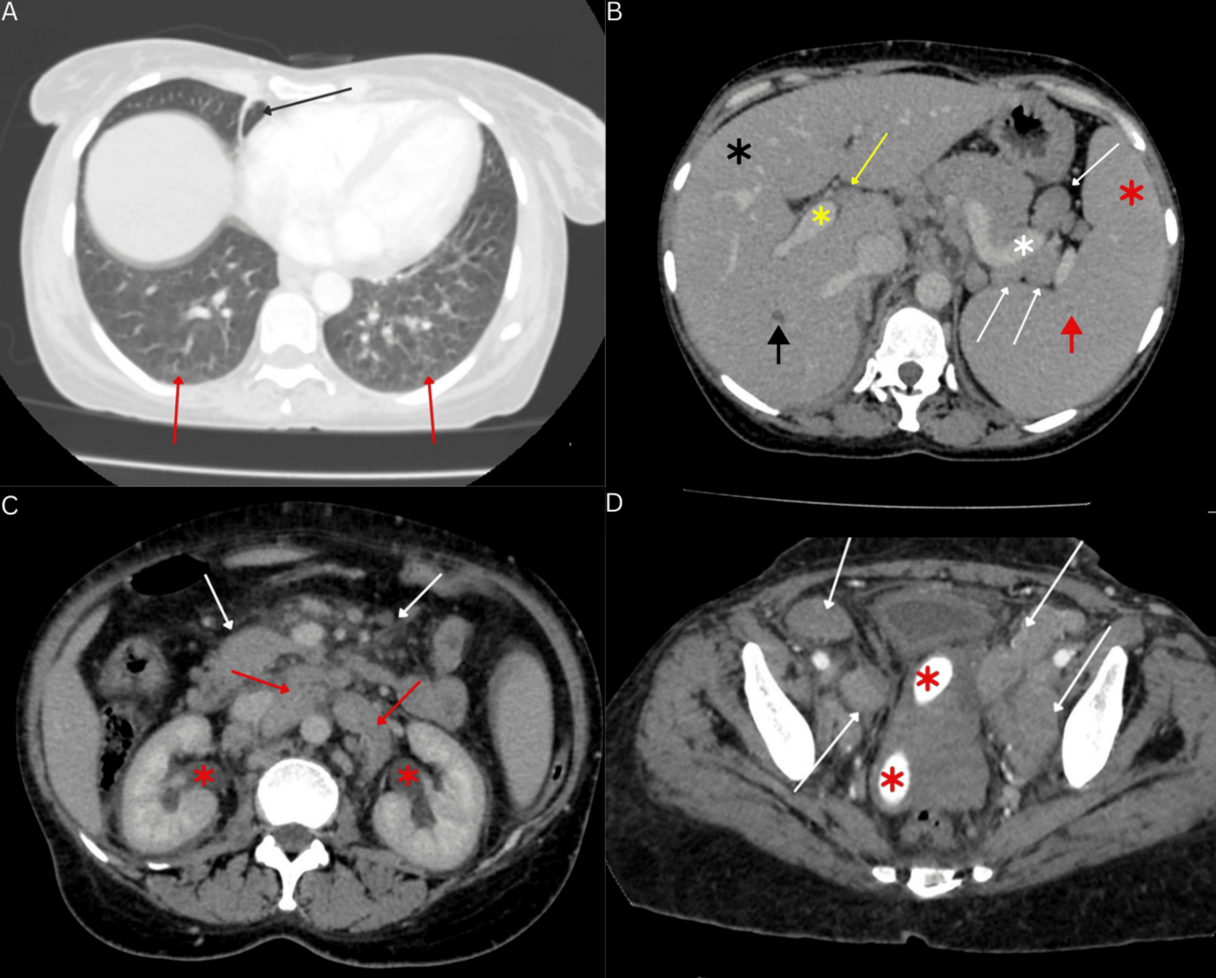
Contrast enhanced axial CT images A: CT chest with lung window demonstrates right lower lobe fibrotic band (black arrow) and diffuse smooth interlobular septal thickening (red arrows). B: Abdominal CT demonstrates hepatomegaly (black asterisk), hepatic nodule (black arrow), porta hepatis lymphadenopathy (yellow arrow), dilated portal vein (yellow asterisk), splenomegaly (red asterisk), splenic nodule (red arrow), splenic hilar lymphadenopathy (white arrow) and splenic vein dilatation (white asterisk). C: Abdominal CT demonstrates bilateral hydronephrosis (red asterisk), paraaortic (red arrows) and mesenteric lymphadenopathy (white arrows). D: Pelvic CT demonstrates bilateral internal and external iliac lymphadenopathy (white arrows) as well as an incidental uterine pessary (red asterisk) CT: computed tomography
